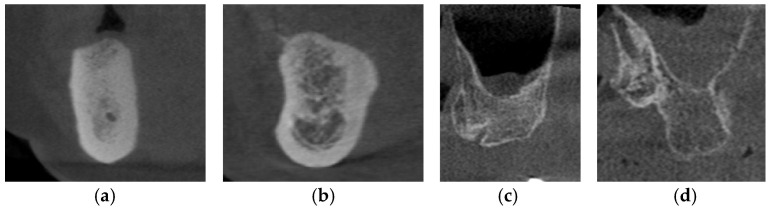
The CBCT images depict the various alveolar bone classifications: (a) Type I; (b) Type II; (c) Type III; and (d) Type IV.
